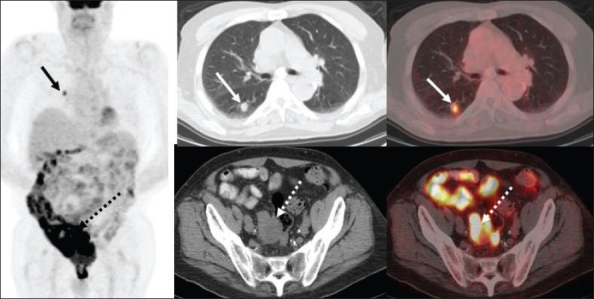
Axial CT and fused PET/CT images at the subcarinal level (top middle and right) and through the pelvis (bottom middle and right), with an MIP image (on left), from a PET study of a 67-year-old man being evaluated for a solitary lung nodule. The right lung nodule shows intense FDG uptake (arrows) consistent with a malignant etiology. Also noted is significant FDG uptake in the cecum and rectosigmoid colon (dotted arrows), for which a colonoscopic evaluation was recommended. The patient was subsequently found to have a large ulcerated rectosigmoid colon adenocarcinoma with tubular adenomas and pan-diverticular disease elsewhere in the colon. After resecting the colon cancer, the solitary lung nodule was also resected and found to be metastatic.
